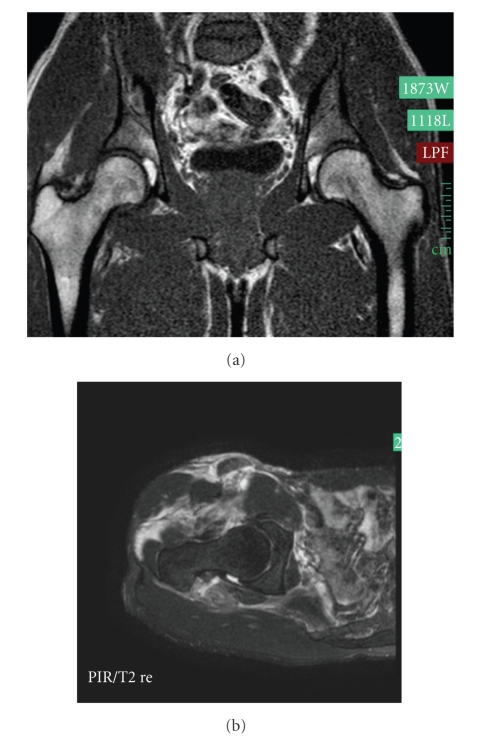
MRI of both hips (a) and transverse cut of the right hip (b) shows no effusion of the congruent right hip, but swelling of the right pelvic muscles.
